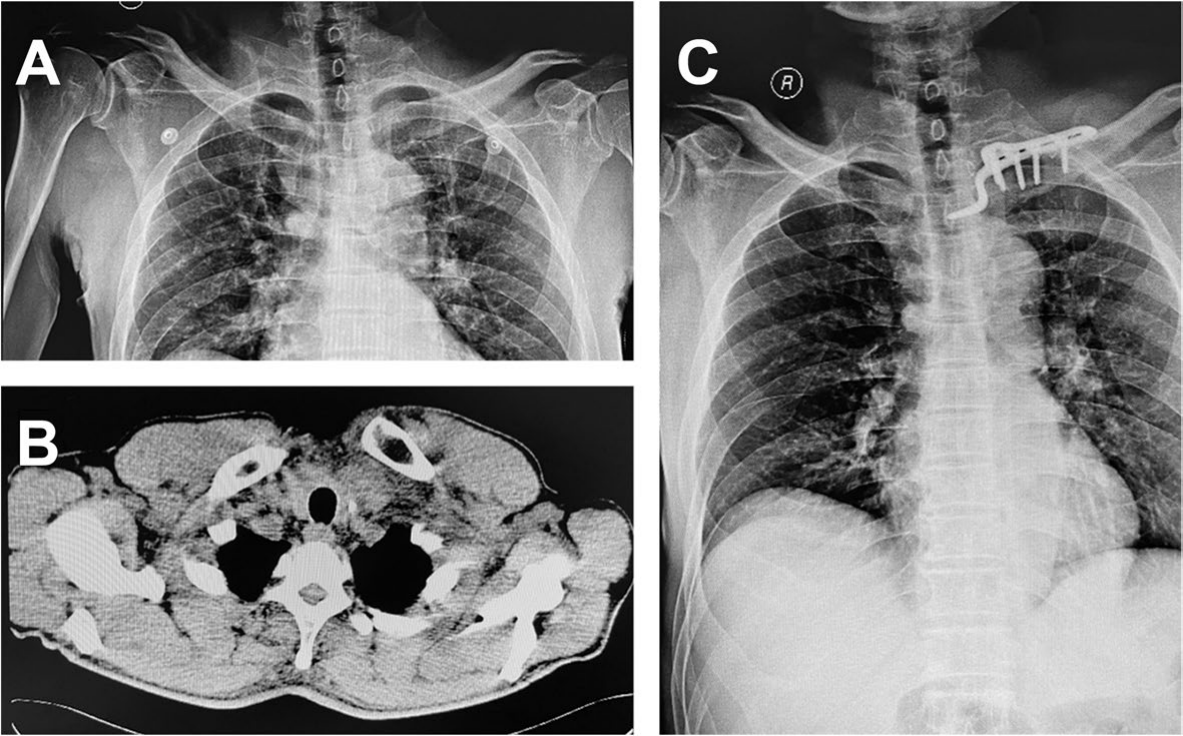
Left anterior sternoclavicular joint dislocation treated with an acromioclavicular joint hook plate (AJHP). A The preoperative radiograph of the patient. B The preoperative computed tomography (CT) scan shows dislocation of the left anterior sternoclavicular joint. C The postoperative radiograph of the patient shows good reduction of the left sternoclavicular joint
